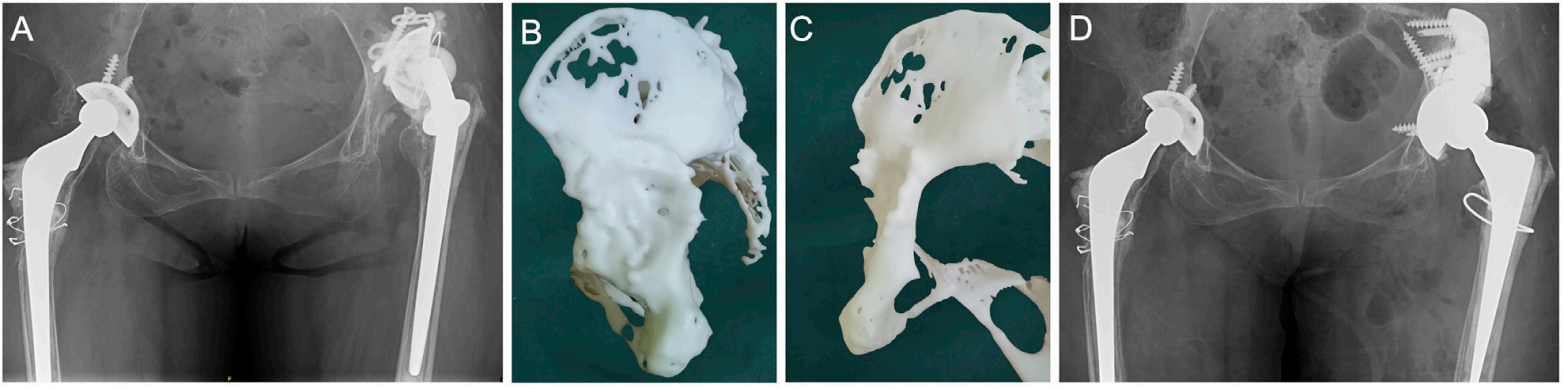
A representative patient receiving this combined therapy. (A) Preoperative AP pelvic x-ray of this patient. (B,C) Rapid prototype reconstruction of this patient’s acetabulum. (D) Postoperative AP pelvic x-ray taken 3 years after revision surgery. Reproduced with permission from authors (Zhang et al., 2022).
